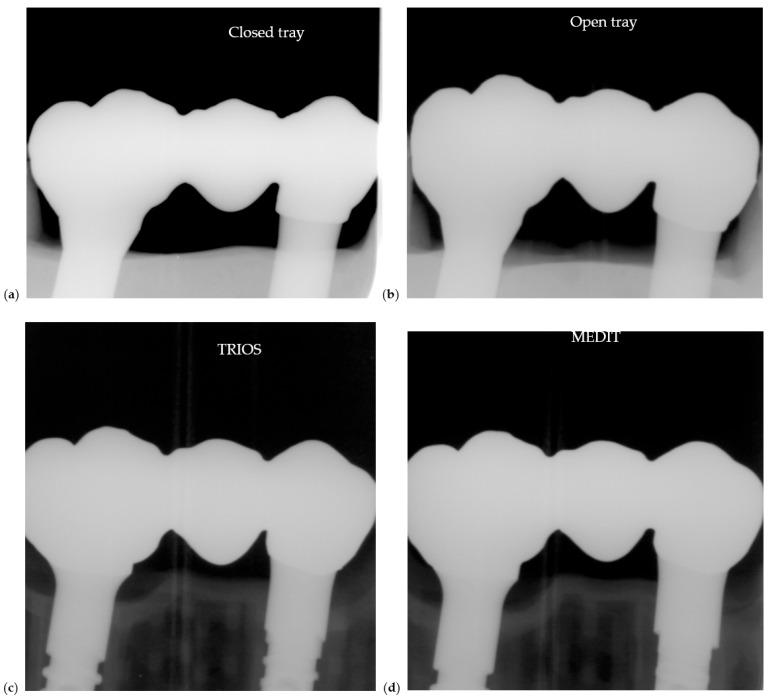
Radiographic evaluation of Zirconia restorations seated on models fabricated with different impression techniques: (a) closed tray conventional impression, (b) open tray impression, (c) TRIOS scan and (d) MEDIT scan.
